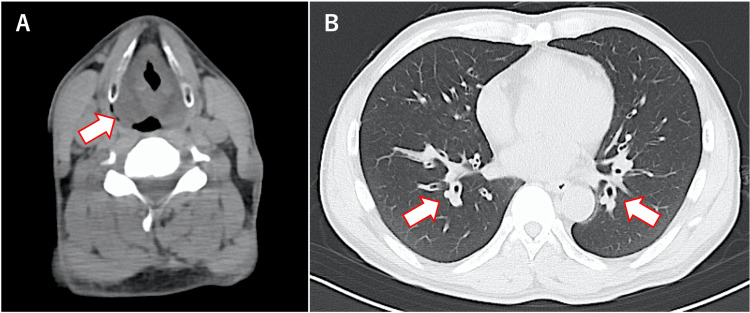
Computed tomography image showing laryngeal edema (A) and diffuse thickening of the bronchial wall (B)
